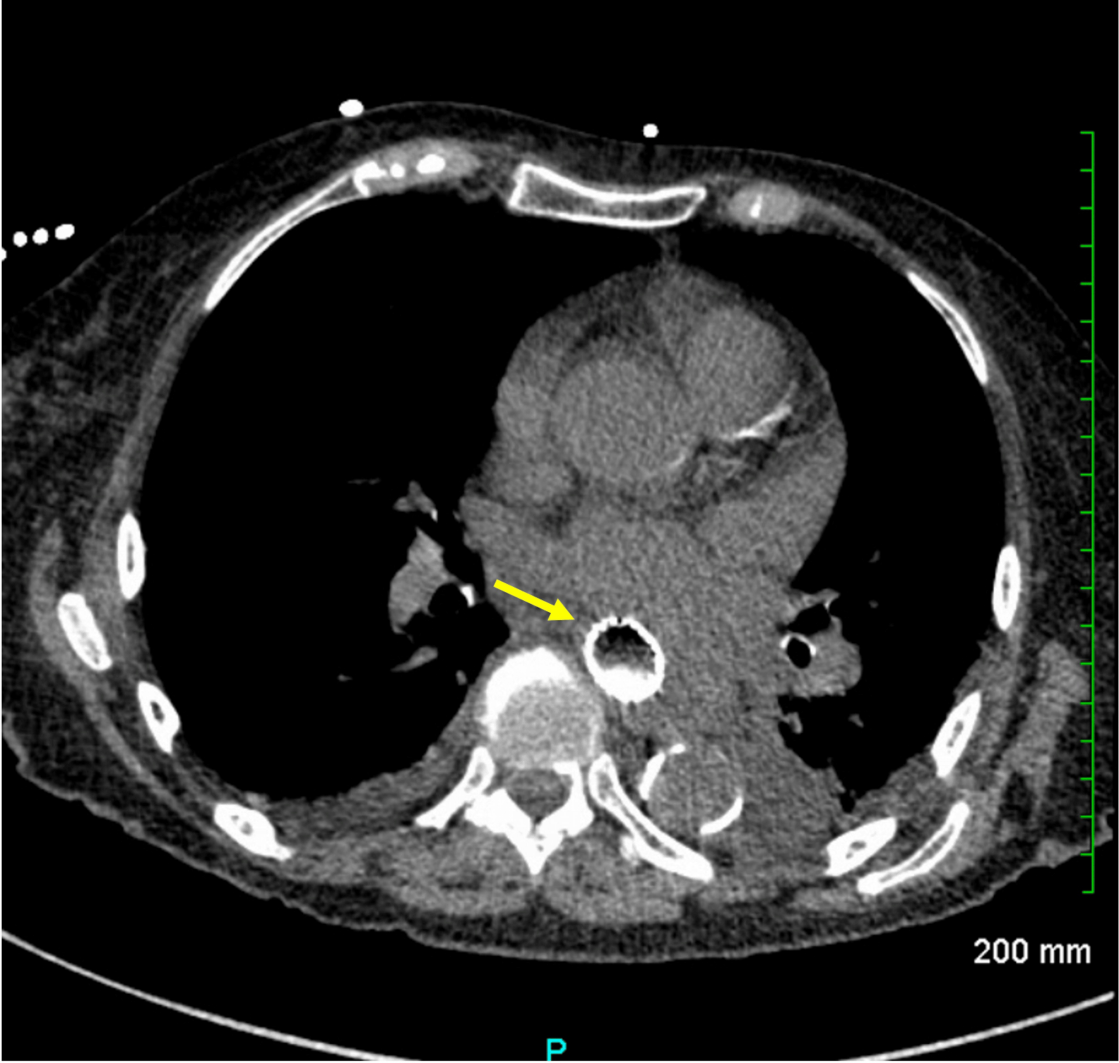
CT of the Chest Without IV Contrast Demonstrating Esophageal Stent In Situ Following Stent Placement Yellow arrow: an esophageal stent is present. This extends from the level just above the carina through the gastric esophageal junction IV: intravenous
